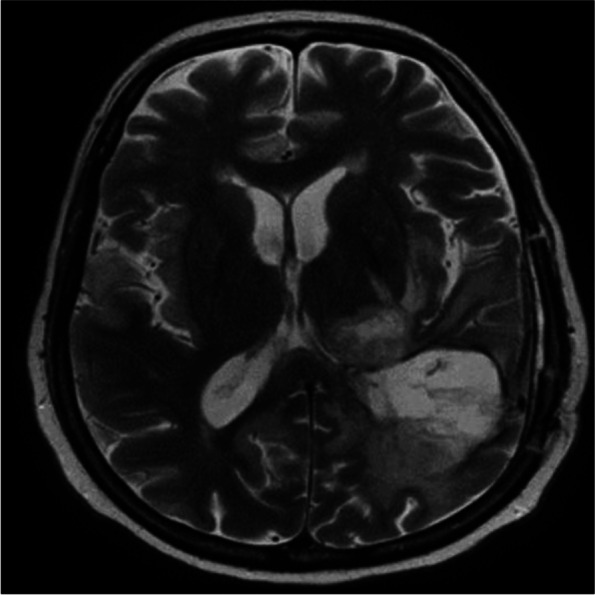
Upon analyzing the patient's brain MRI, increased hyperintensity and diffusion restriction signals were observed in several areas, including the right temporal lobe, right mesial temporal structure, insular cortex, inferior frontal lobe, and cingulate gyrus, leading to suspicions of viral encephalitis
